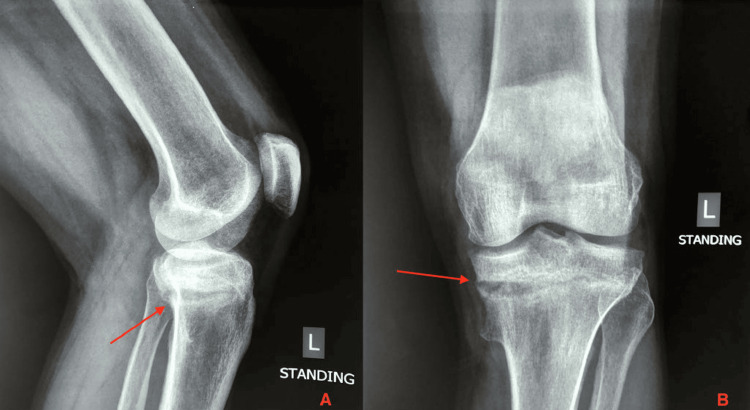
Left knee lateral (A) and AP (B) X-ray. Proximal tibia fracture was also present upon presentation.
